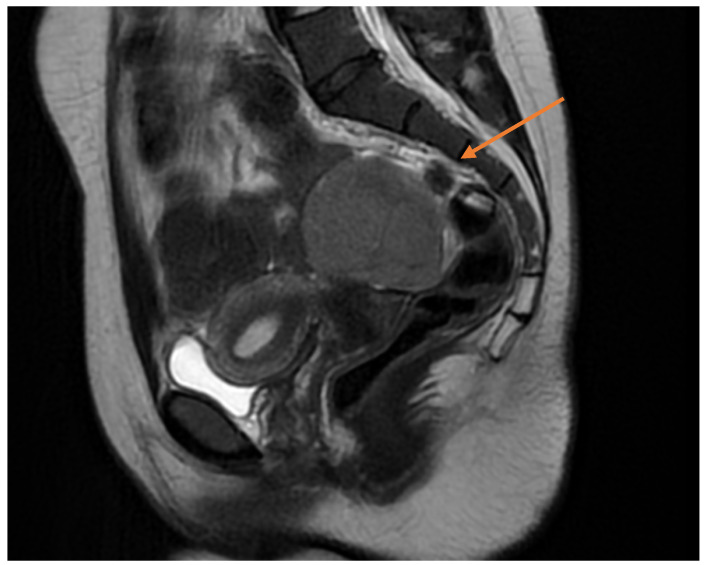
Sagittal pelvic MRI (T2-weighted) demonstrating a septated adnexal mass in the pouch of Douglas, with cystic and nodular components suggestive of intratumoral hemorrhage (yellow arrow).
